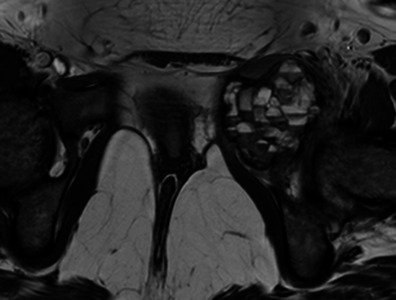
Transverse T2-weighted MRI: aneurysmal bone cyst of the left pubic branch, with multiple small fluid-fluid levels
